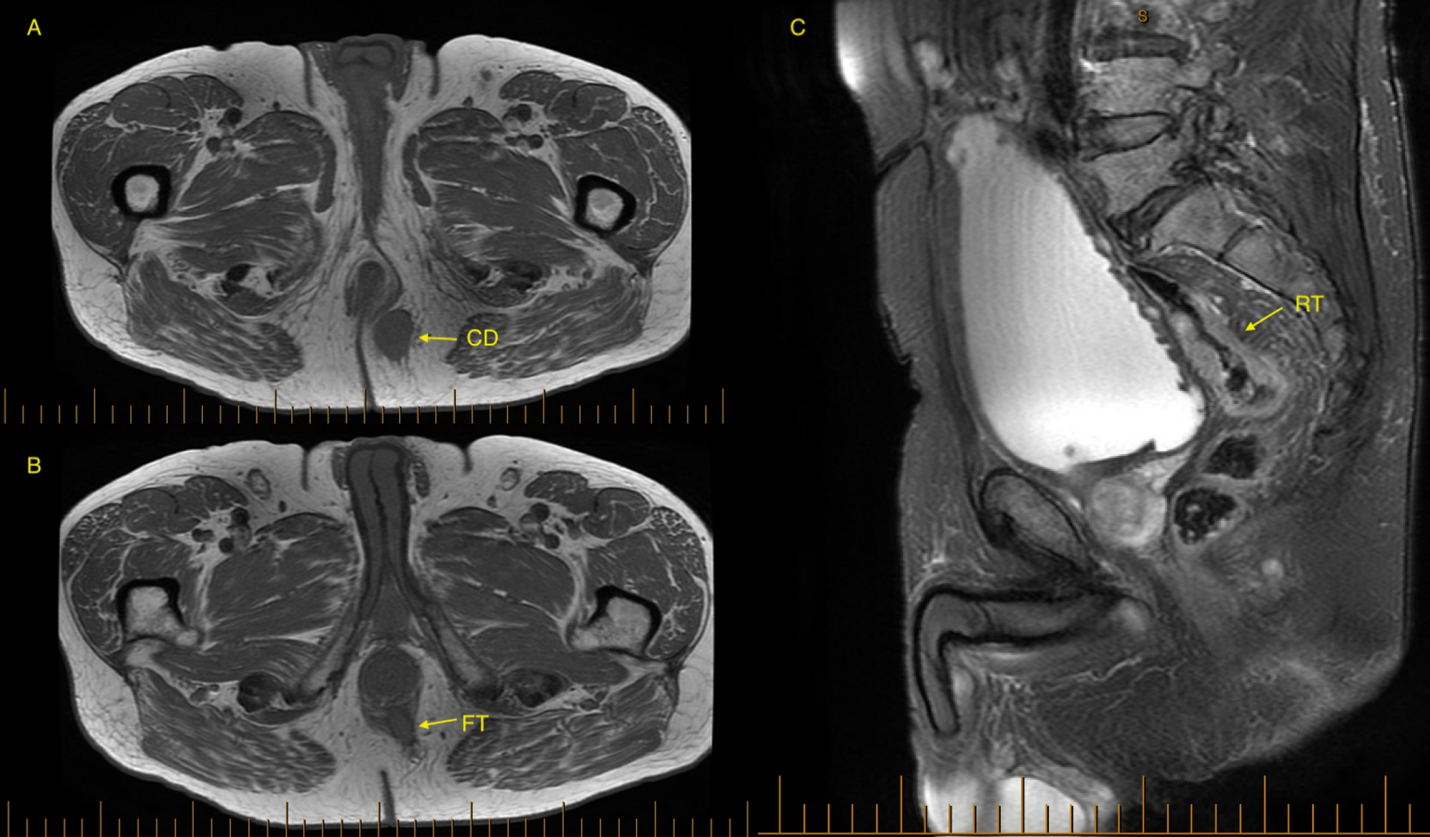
Axial and saggital views of MRI demonstrate cutaneous metastatic deposit (A), fistula-in-ano (B) and upper rectal tumor (C). CD, cutaneous deposit; FT, fistula tract; RT, rectal tumor
